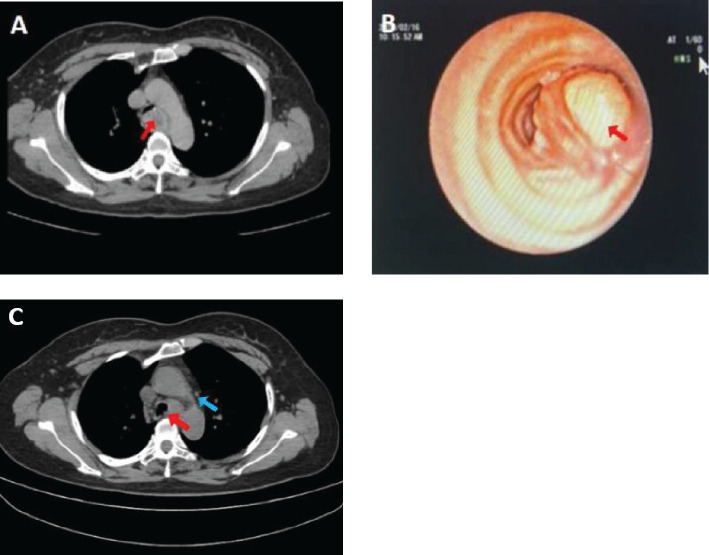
Chest CT scan shows a nodular soft tissue density shadow on the left wall of the lower segment of the trachea, with a maximum diameter of 2.8 cm [(A), arrow]. The bronchoscopic view reveals a mass obstructing the lumen of the anterior basal segment of the lower lobe of the left lung [(B), arrow]. Reexamination with PET-CT scan demonstrates a nodular shadow in the lower trachea, with a maximum diameter of 1.3 cm [(C), red arrow] and multiple enlarged mediastinal lymph nodes (Panel C, blue arrow).
